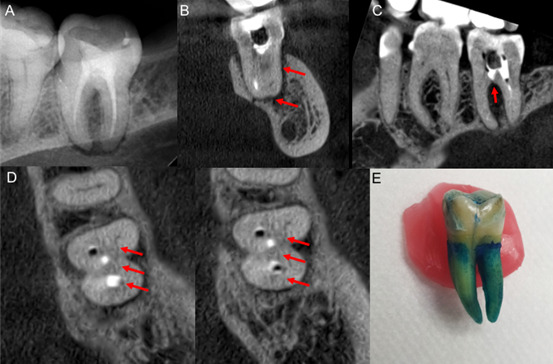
Caso 2. (A) RP permite observar la imagen radiolúcida de la lesión periapical y furca y la obturación de los conductos radiculares. Cortes coronal y sagital (B y C): el sellado hiperdenso parcial de los conductos, defecto óseo con separación de la tabla ósea vestibular y el compromiso de la región de furca. Cortes axiales (D) observación de las líneas de fractura mesiodistal y vestibular. (E) fotografïa clínica que evedencia la fractura longitudinal corono-radicular.
